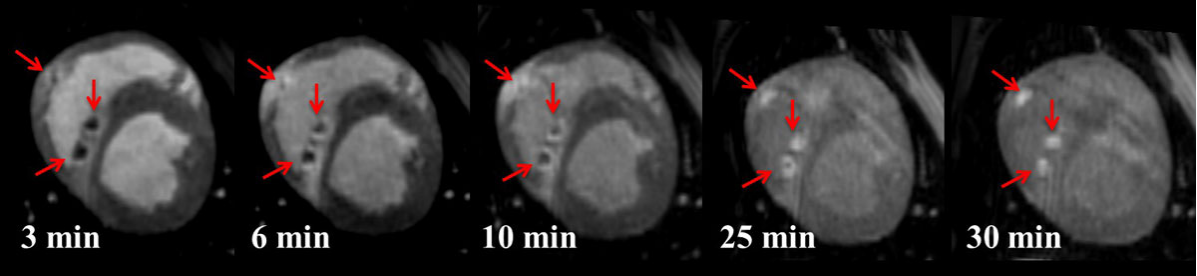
Example of DCE-MRI of acute ventricular lesions. Red arrows indicate the lesions.
